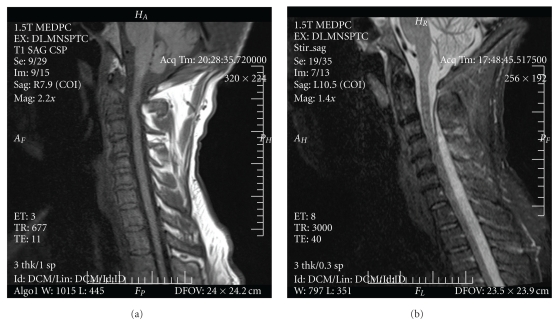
Saggittal T1 cervical spine MRI: day 1 (a) and day 11 (b). Serial MRI images of the cervical spine from hospitalization day 1 (a) and day 11 (b). (a) shows a relatively normal cervical spine with only mild degenerative disc protrusion and mild canal stenosis at C6-C7. (b) shows epidural enhancement and marked anterior compression of the spinal cord beginning at the level of C1 and continuing caudally.
